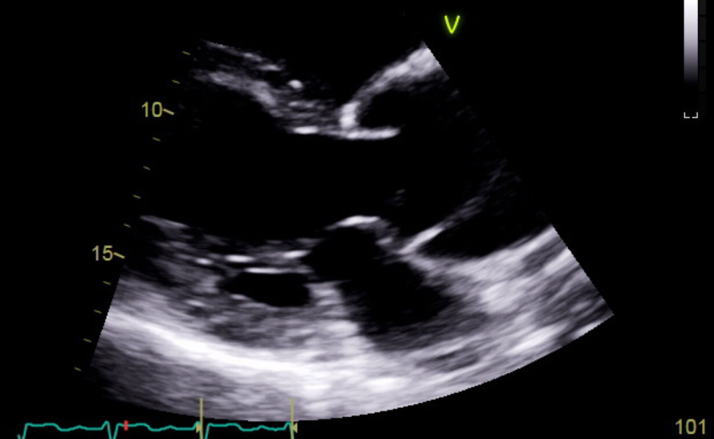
Transthoracic Echocardiogram: Parasternal Long-Axis View TTE demonstrating dissection flap in the ascending aorta, above the aortic valve leaflets. Dilation of ascending aorta is visualized as well. TTE = transthoracic echocardiography.
